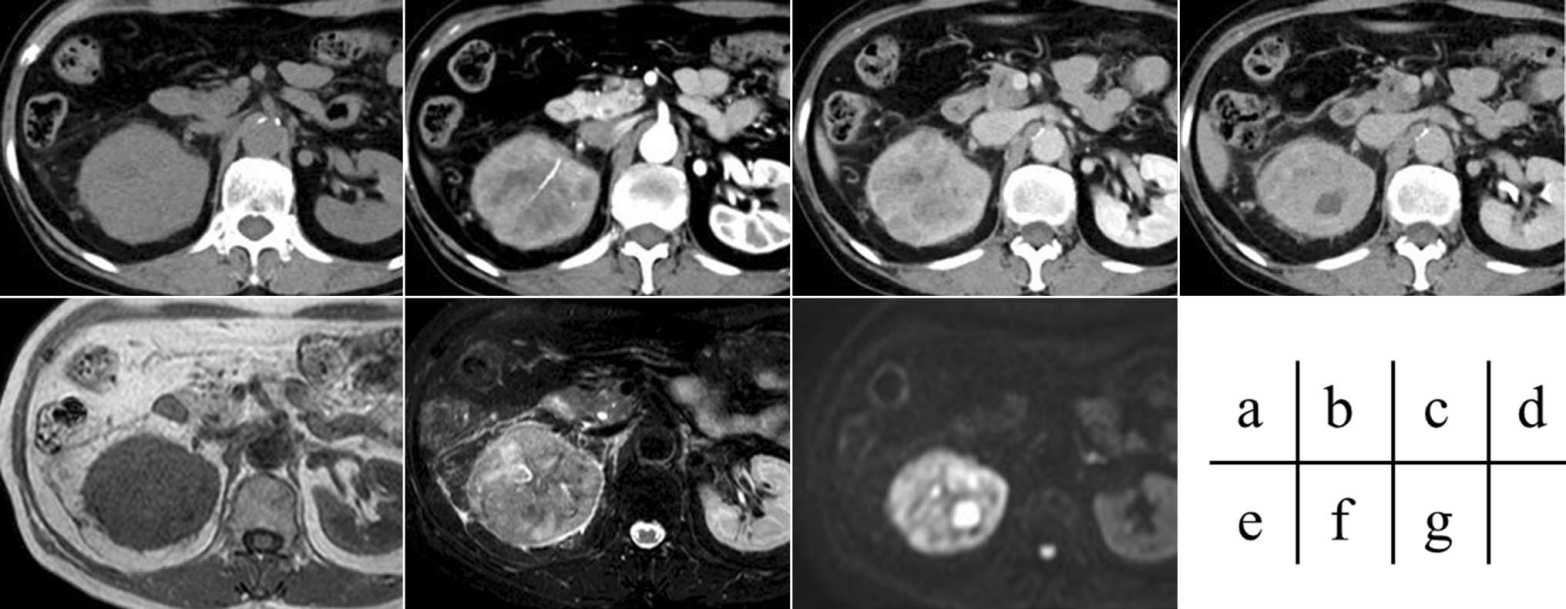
Images from an 82-year-old woman with unclassified RCC. A large mass lesion with invasive growth is apparent in the right kidney. The tumor is isodense on unenhanced CT (a) and exhibits a progressive pattern on DCE imaging (b–d). The tumor appears isointense on T1-weighted imaging (e), hypo- to hyperintense with heterogeneous internal appearance on T2-weighted imaging (f) and hyperintense on DWI (g), with an evident cystic component. Unclassified RCC was diagnosed after surgery. Recurrence was confirmed 329 days after surgery
